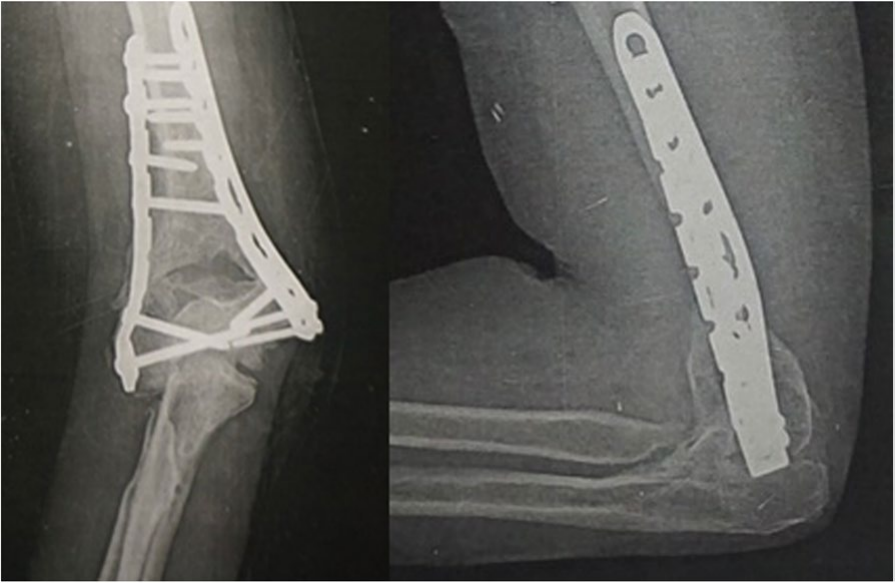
Follow up X-ray for the same patient (case #14) 9 months after spanning plate removal showing good union
